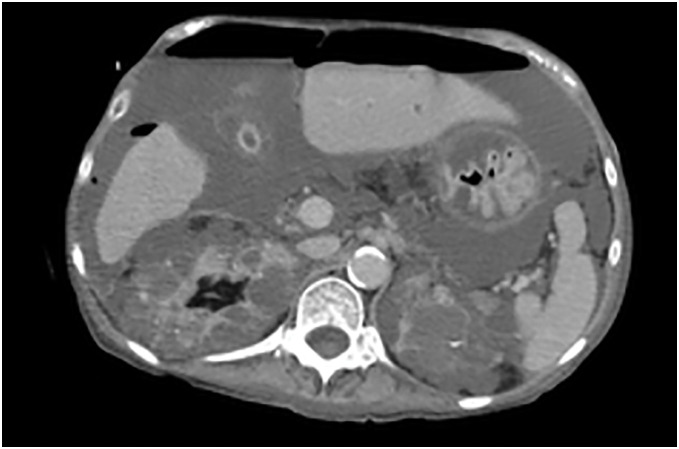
Free air was observed in the abdominal cavity on CT.
